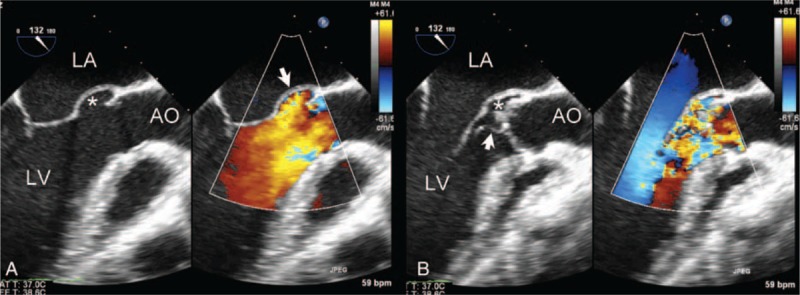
Two-dimensional color Doppler transesophageal echocardiography showing P-MAIVF in the left ventricular outflow tract long-axis view. (A) Two-dimensional TEE showing P-MAIVF expanded (white star) and color Doppler imaging showing a flow filling the cavity with intact wall in systole. (B) P-MAIVF collapsed (white star), aortic vegetation (arrow), and severe aortic regurgitation in diastole. AO = aorta; LA = left atrium; LV = left ventricle; P-MAIVF = pseudoaneurysm of the mitral-aortic intervalvular fibrosa; TEE = three-dimensional transesophageal echocardiography.
